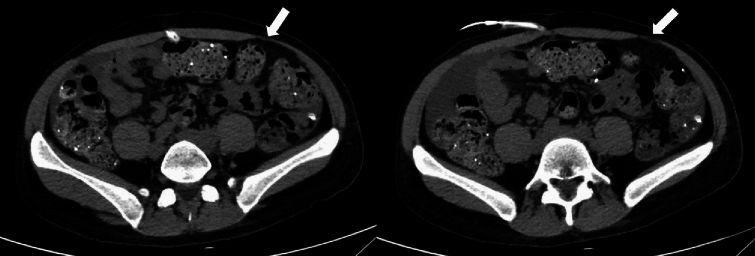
Abdominal CT demonstrated thinning of the left external oblique and internal oblique muscles (arrows). Abdominal masses and true abdominal hernias were excluded
